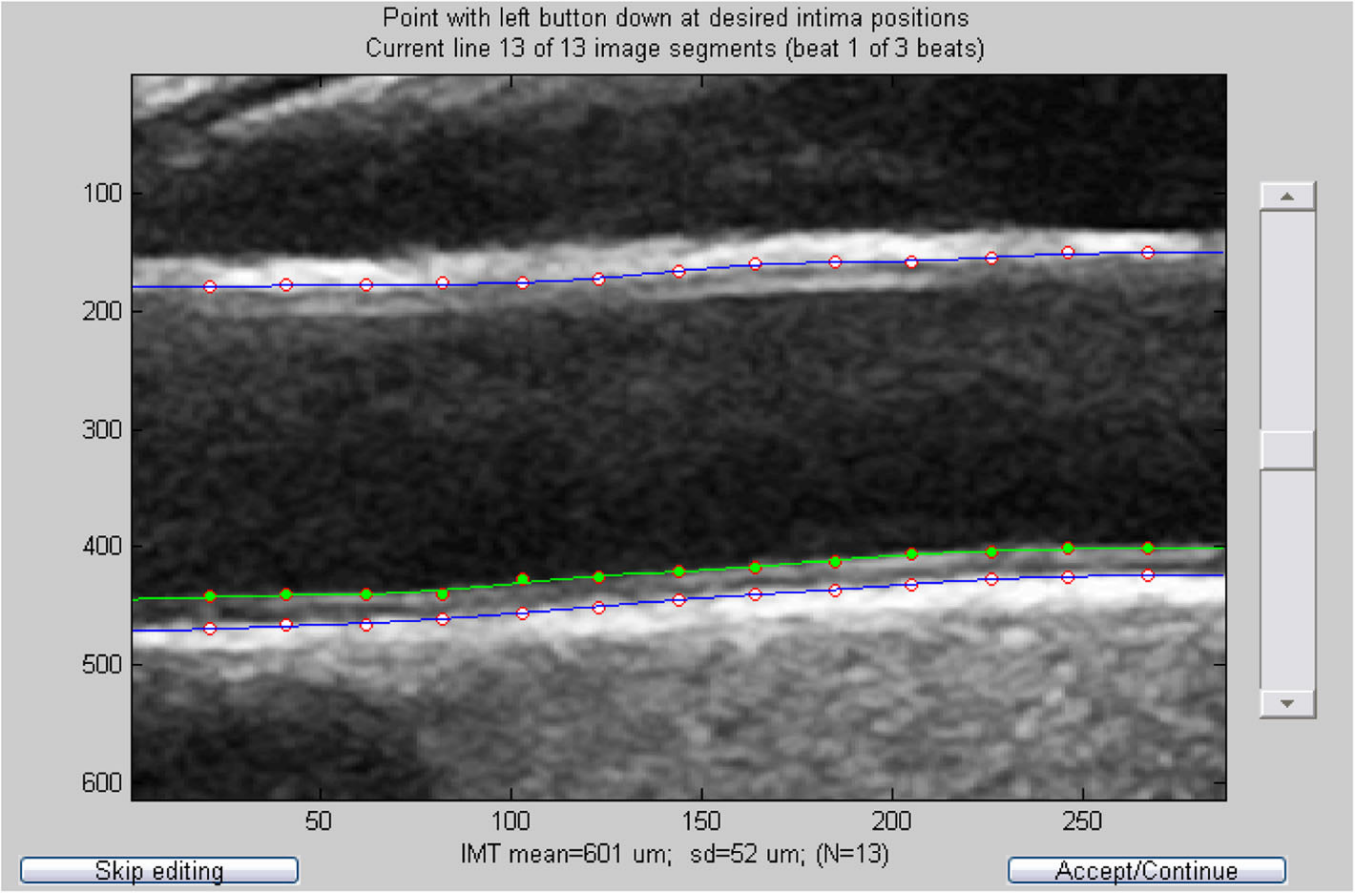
Automatic analysis of the common carotid artery distention and cIMT made with edge wall tracking of ultrasound B-mode recordings. The media-adventitia boundaries are defined by the blue lines and the distance between them is the carotid diameter. The lumen-intima boundary is shown as the green line and defines the cIMT on the far wall together with the blue line
